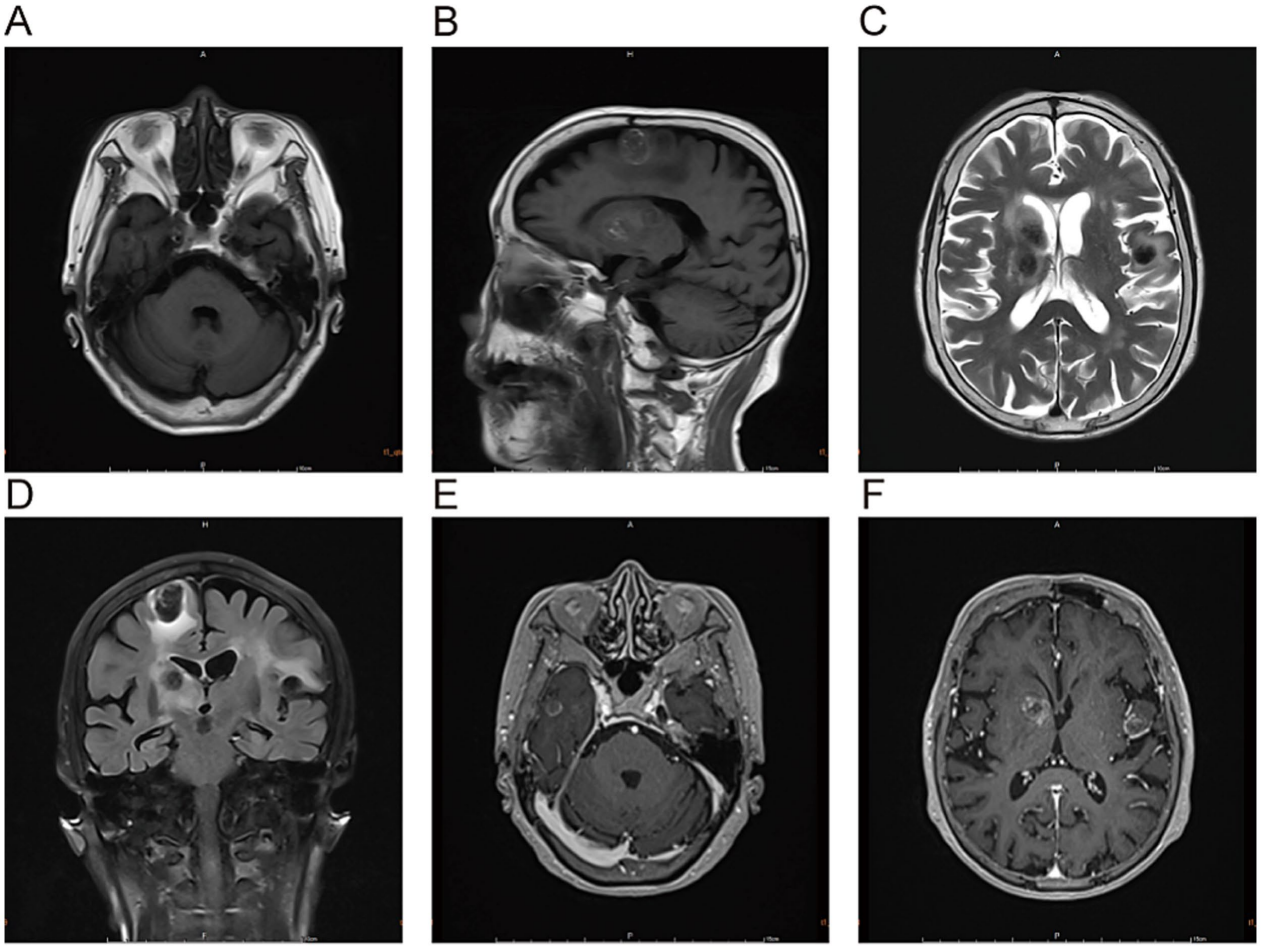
The lesions in the right frontal-temporal lobe and basal ganglia region, as well as the left frontal lobe, predominantly exhibit high signal intensity on T1-weighted images (A,B) and low signal intensity on T2-weighted images (C). T2-FLAIRE sequences (D) reveal patchy perilesional edema surrounding the lesions. Contrast-enhanced scans (E,F) demonstrate ring enhancement.
